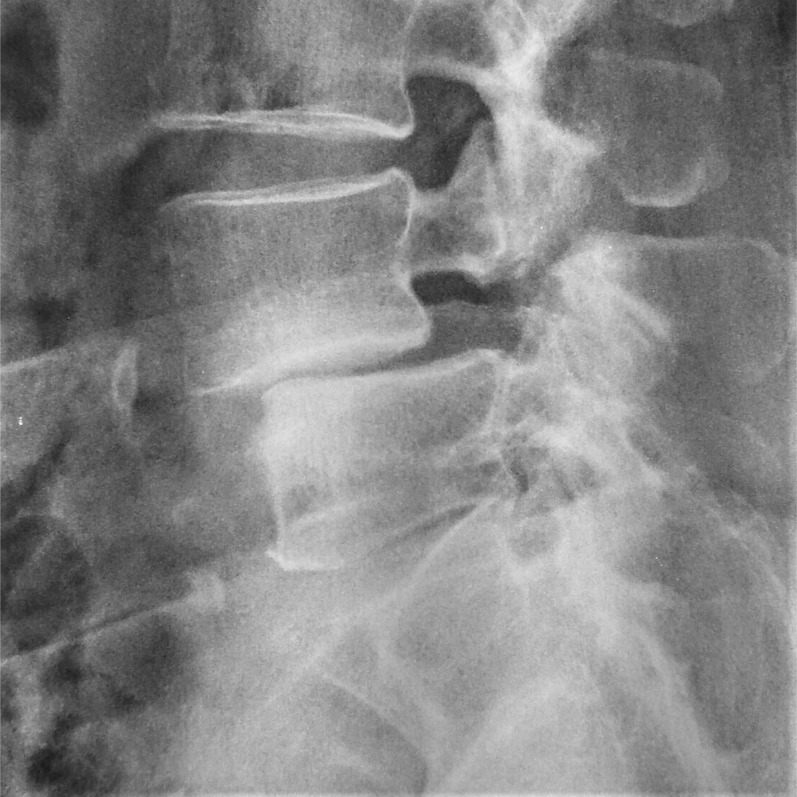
Profile X-ray of a patient with grade I-II spondylolisthesis of the L4 vertebra.
